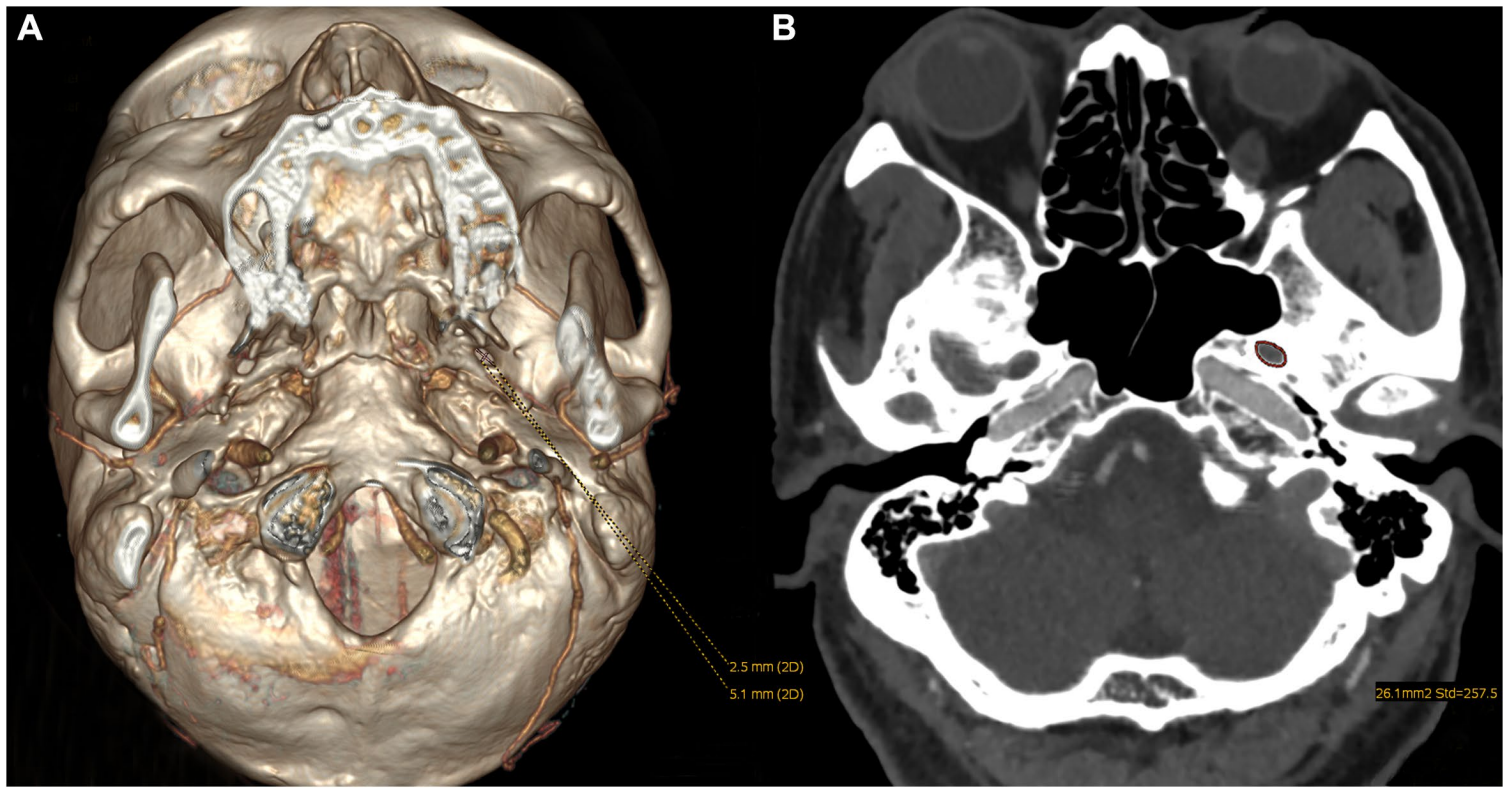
The parameters of an FO measured on CT images: (A) the width and length were measured on a 3D reconstruction image and (B) the area was measured on the axial image.
